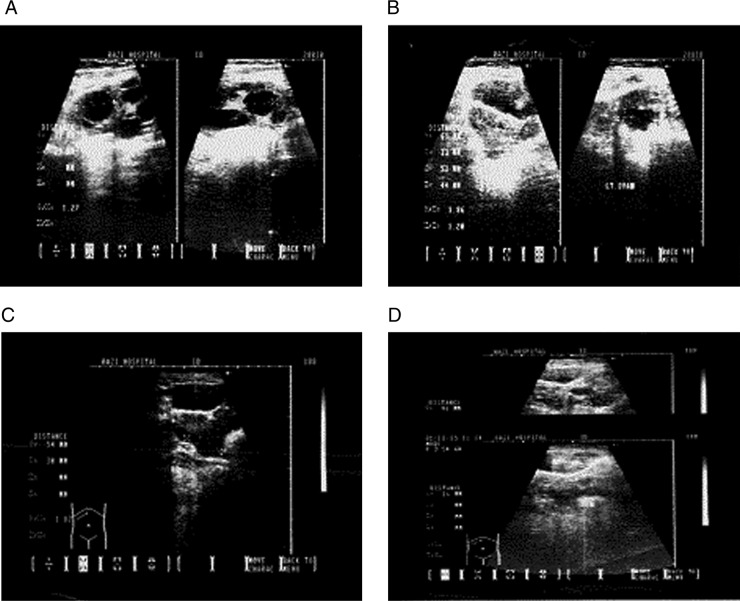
Follow-up abdominal sonography showed significant ovarian volume and cyst regression within 4 months of levothyroxine therapy (A) after 2 months, (B) after 4 months, (C) after 8 months (right ovary), and (D) after 8 months (left ovary).
